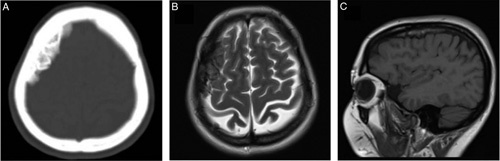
(A–C) A computed tomography scan showing an extra-axial calcified lesion in the right frontal lobe near the calvaria (A). MRI studies in T2A (B) and T1A (C) show hypointense lesions compatible with calcification/ossification.
